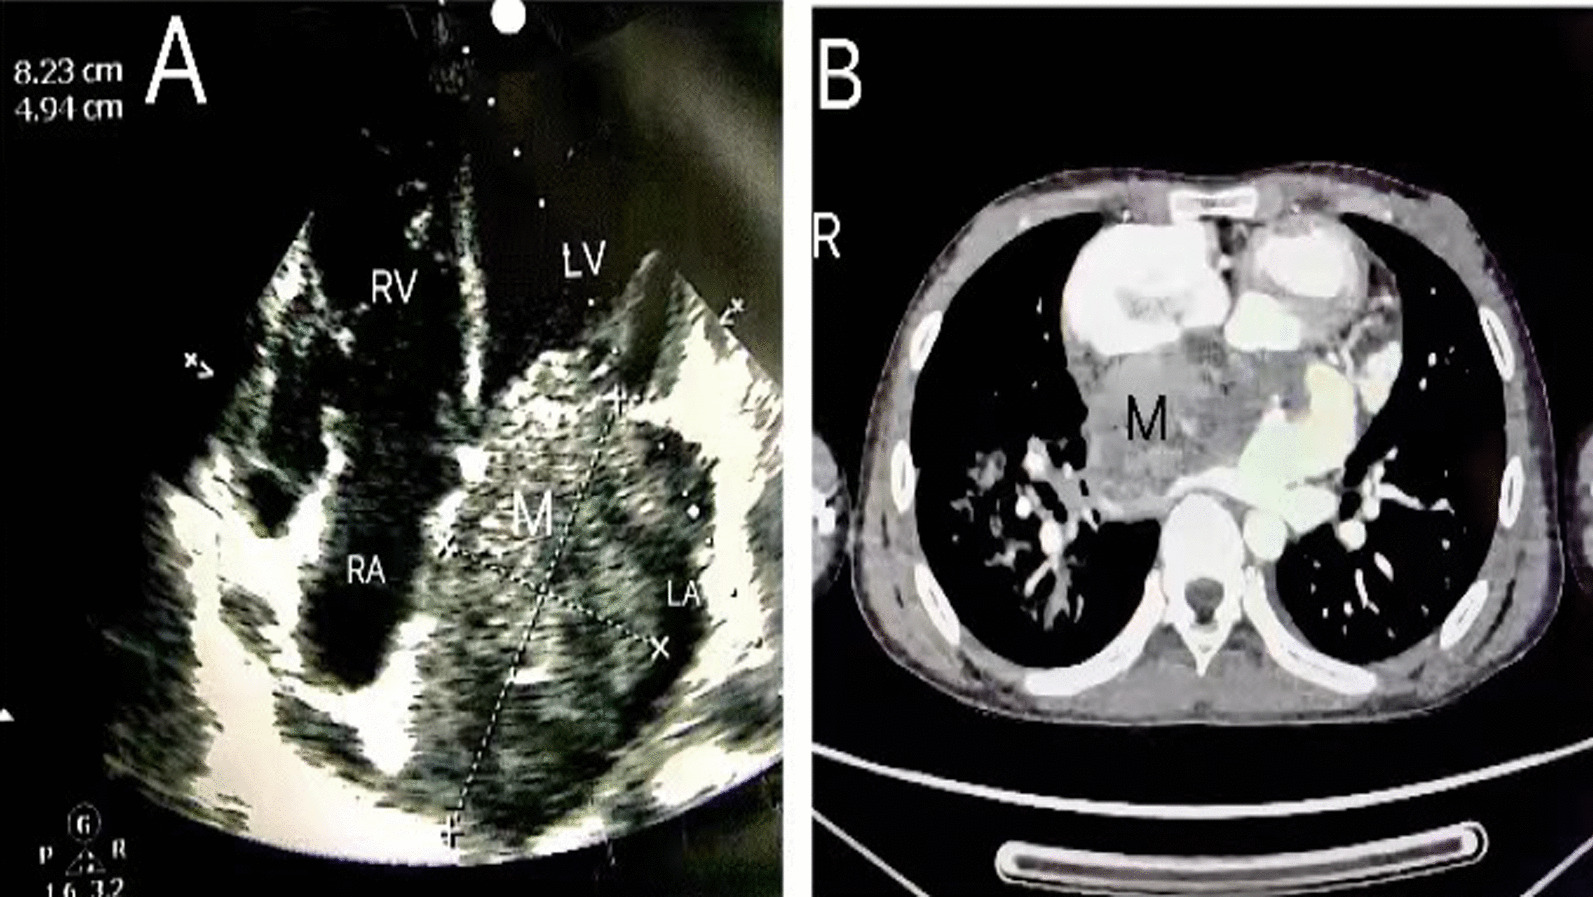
Transthoracic echocardiogram (the apical four chamber view) and Cardiac computed tomography. A Transthoracic echocardiogram. B Cardiac computed tomography. *LA* Left atrium, *LV* Left ventricle, *M* Mass. *RA* Right atrium, *RV* Right ventricle
